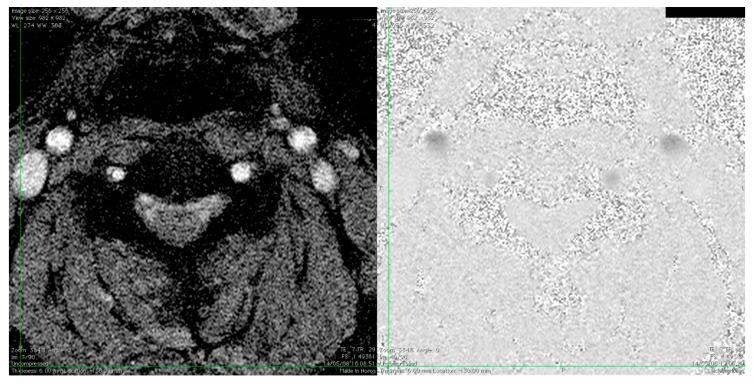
The left image shows a time-of-flight image of the neck in the transverse scan plane. The image is used for identifying and marking the carotid arteries for velocity estimation with phase contrast MRI. The right image shows the phase contrast image using a through-plane sequence. Images were provided by co-author Kristoffer Lindskov Hansen.
